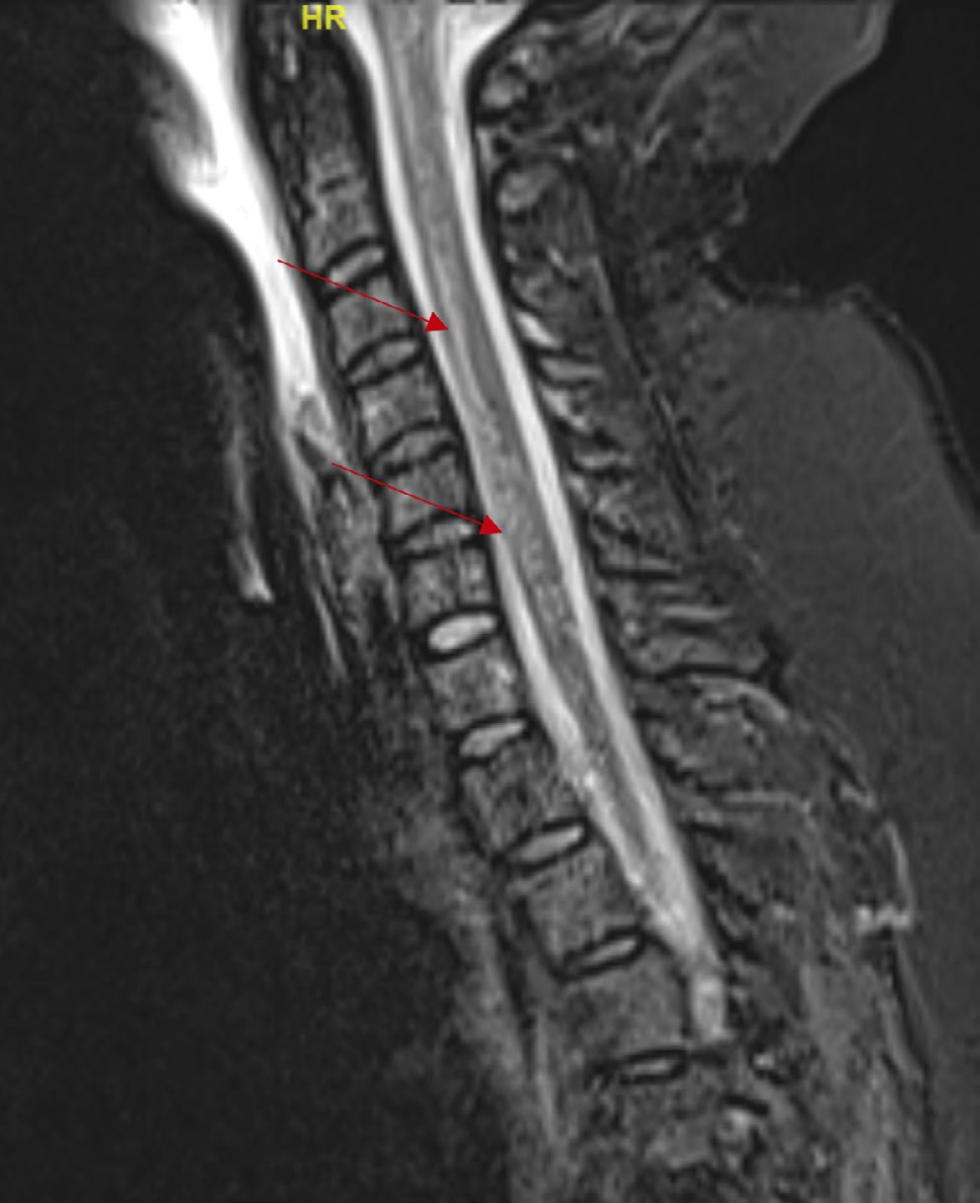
Sagittal T2 MRI abnormality seen within the spinal cord in high cervical area. Red arrows indicate hyperintensity shown on imaging
